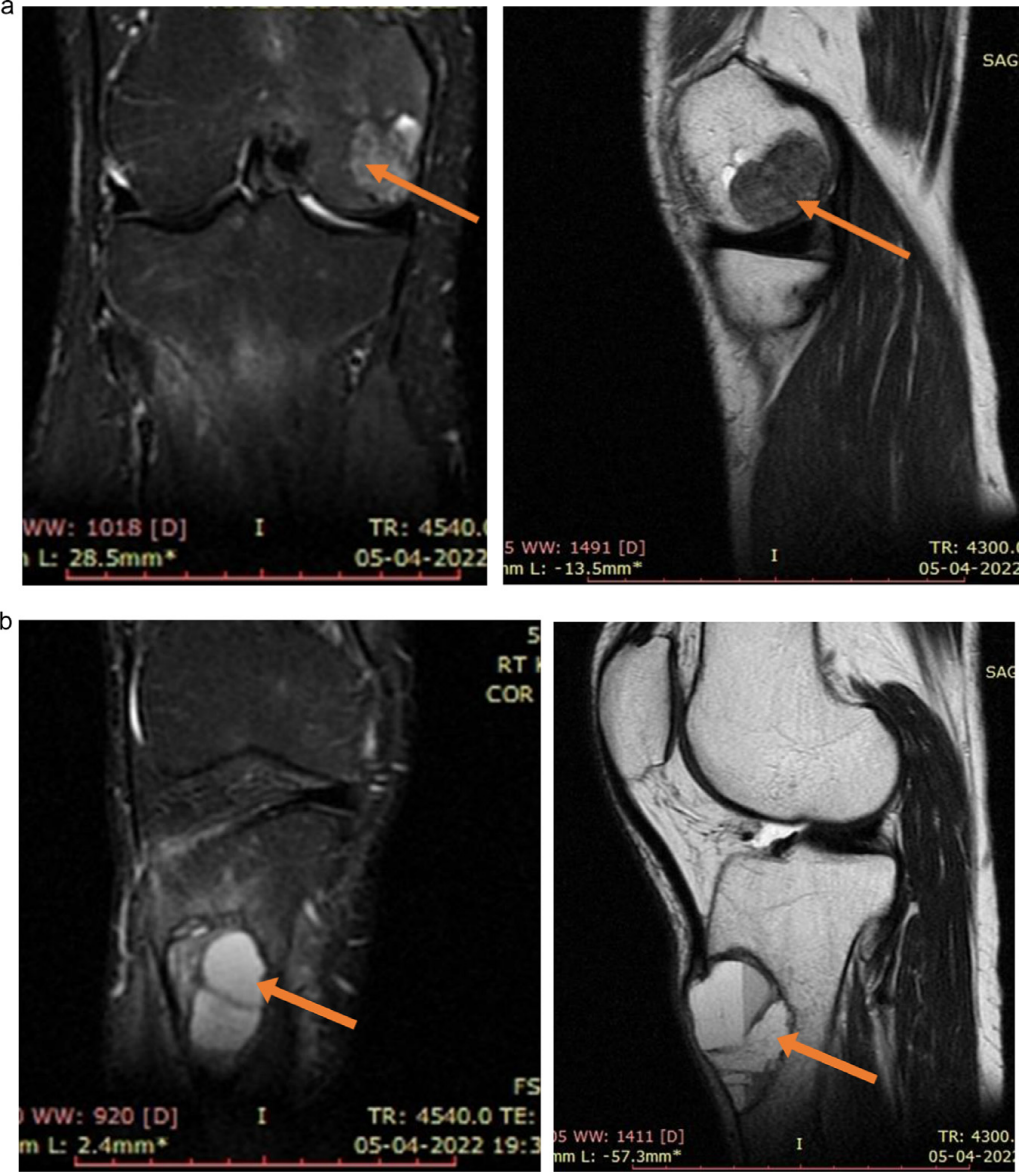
(A) MRI of the right knee shows a well-defined heterogeneously hyper-intense lesion on Short-TI Inversion Recovery and hypo intense on T2 with small T2 hyperintense foci in the epiphyseal region of medial femoral condyle with subarticular extension. (B) Another eccentric multiloculated fluid signal intensity lesion with fluid - fluid levels which are hyper intense on T2 and Short-TI Inversion Recovery noted in the upper end of the tibia with moderate marrow edema.
